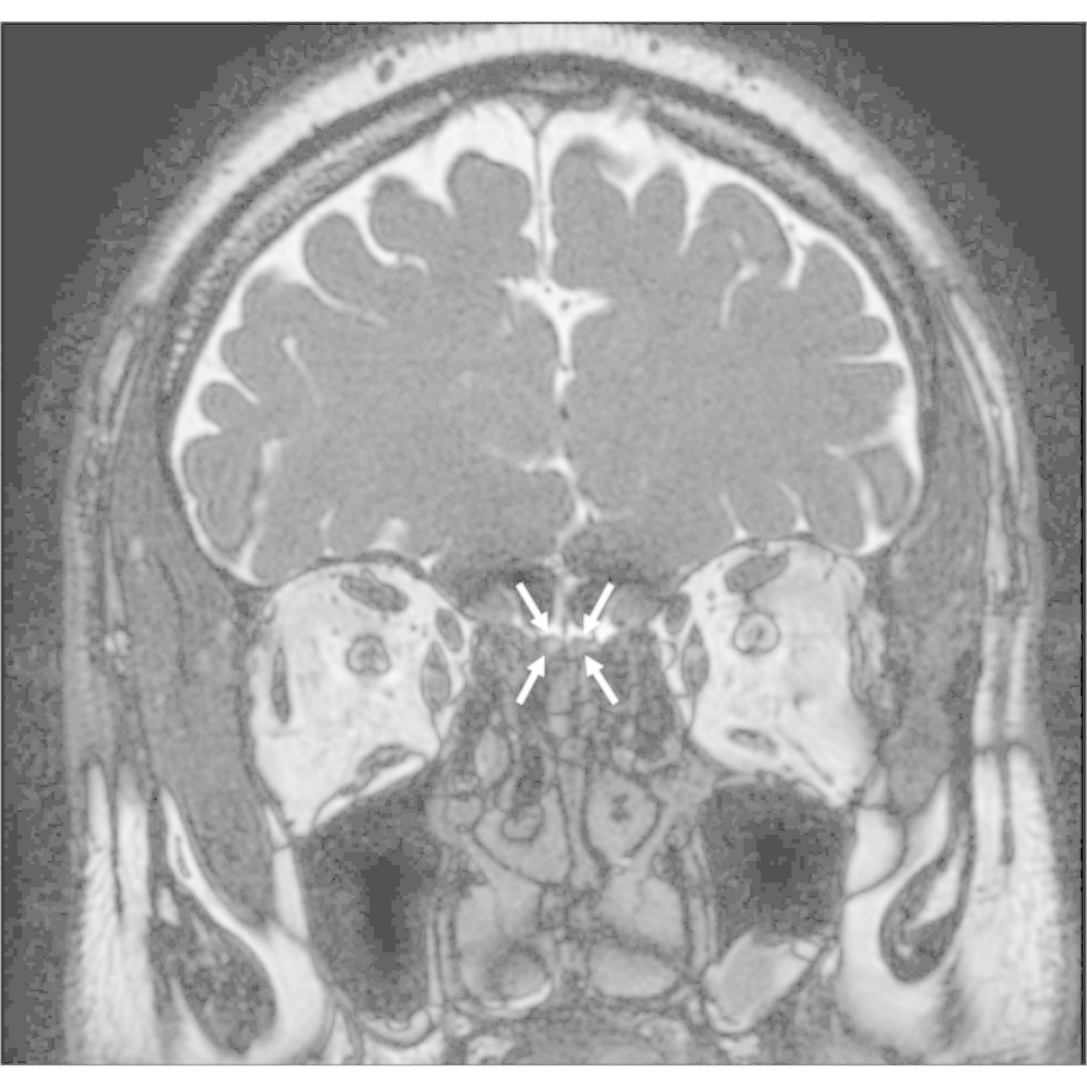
Coronal T2-weighted image Coronal T2-weighted images showing the OBs on both sides with a hypointense ovoid structure (arrow). Note the hyperintense CSF surrounding the OBs. OB: olfactory bulb; CSF: cerebrospinal fluid
